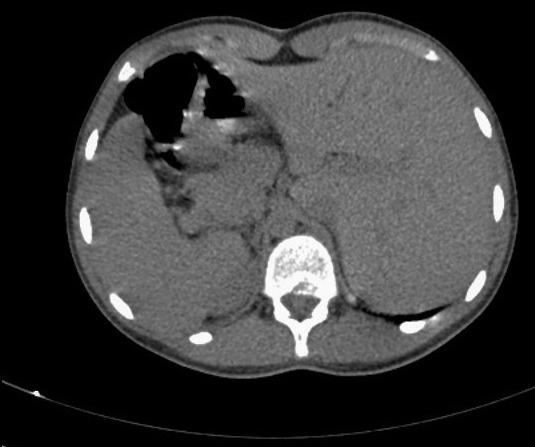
CT abdomen in axial section without contrast injection, shows situs inversus totalis with liver in the left side and spleen in the right side
